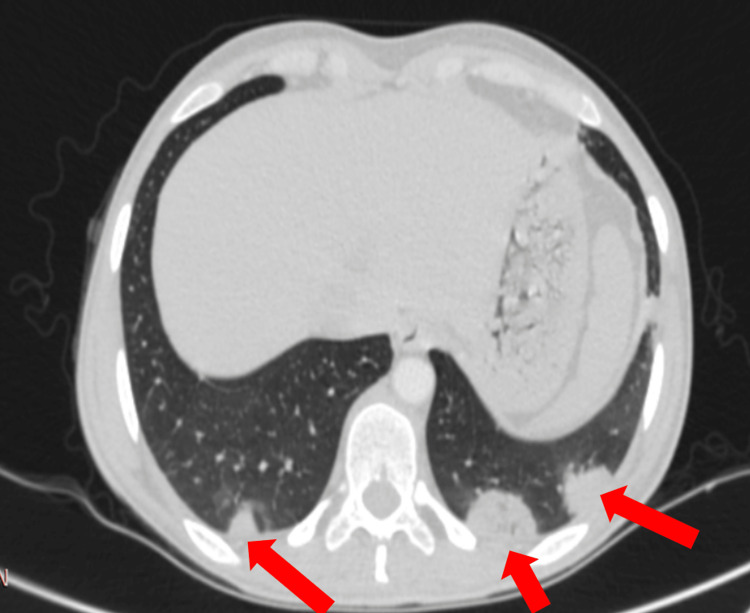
Thorax CT showing consolidated substances related to emboli in the posterior basal segment of the right and left pulmonary lower lobe (red arrows)
